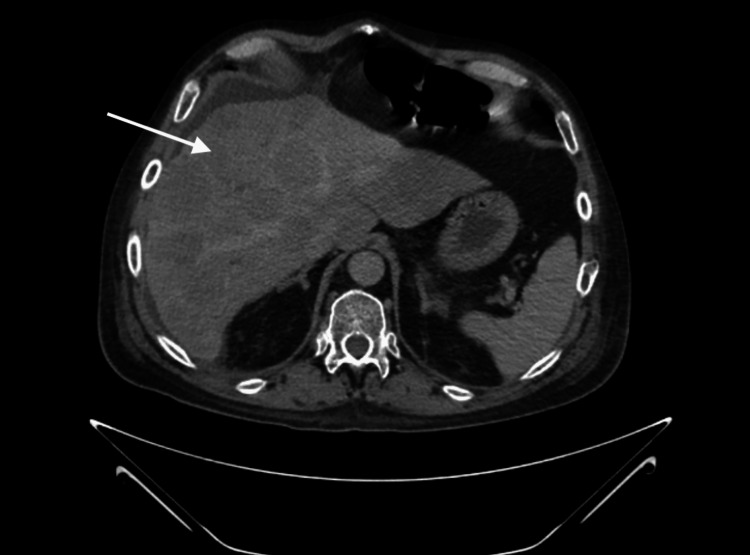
Repeat CT abdomen showing heterogeneous liver with multiple masses concerning for metastasis. Arrow points towards the heterogeneous masses seen in the liver on CT.
